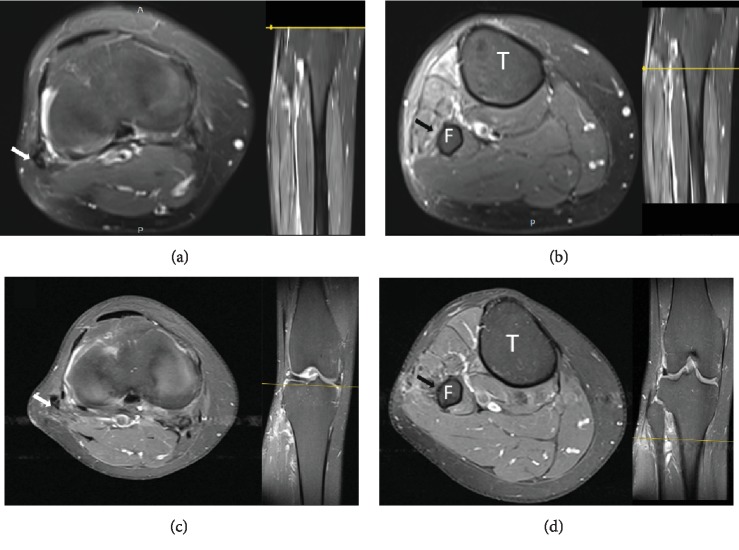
T2-weighted MR axial images of the knee at 6 months (a and b) and 10 months (c and d) postoperative in levels corresponding to preoperative views. a and c are taken at a level above the fibular head. The absence of a high signal is noted in CPN (white arrow). b and d are taken at the level in which a “transverse limb sign” was observed in preoperative MRI (black arrow). T: tibia; F: fibula.
